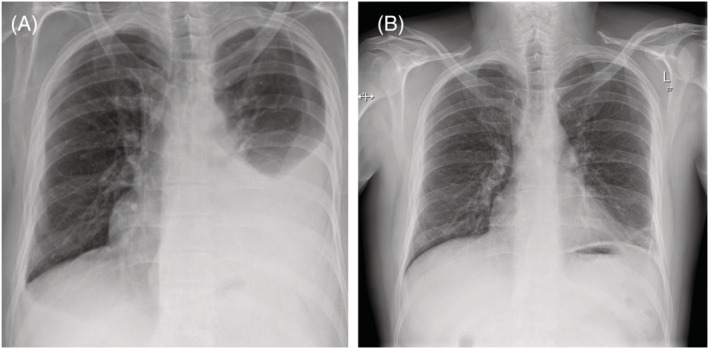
(A) Chest radiograph taken on the seventh day of admission showing large‐volume left‐sided pleural effusion. (B) Chest radiograph taken 3 weeks post‐discharge showing complete resolution of the effusion
